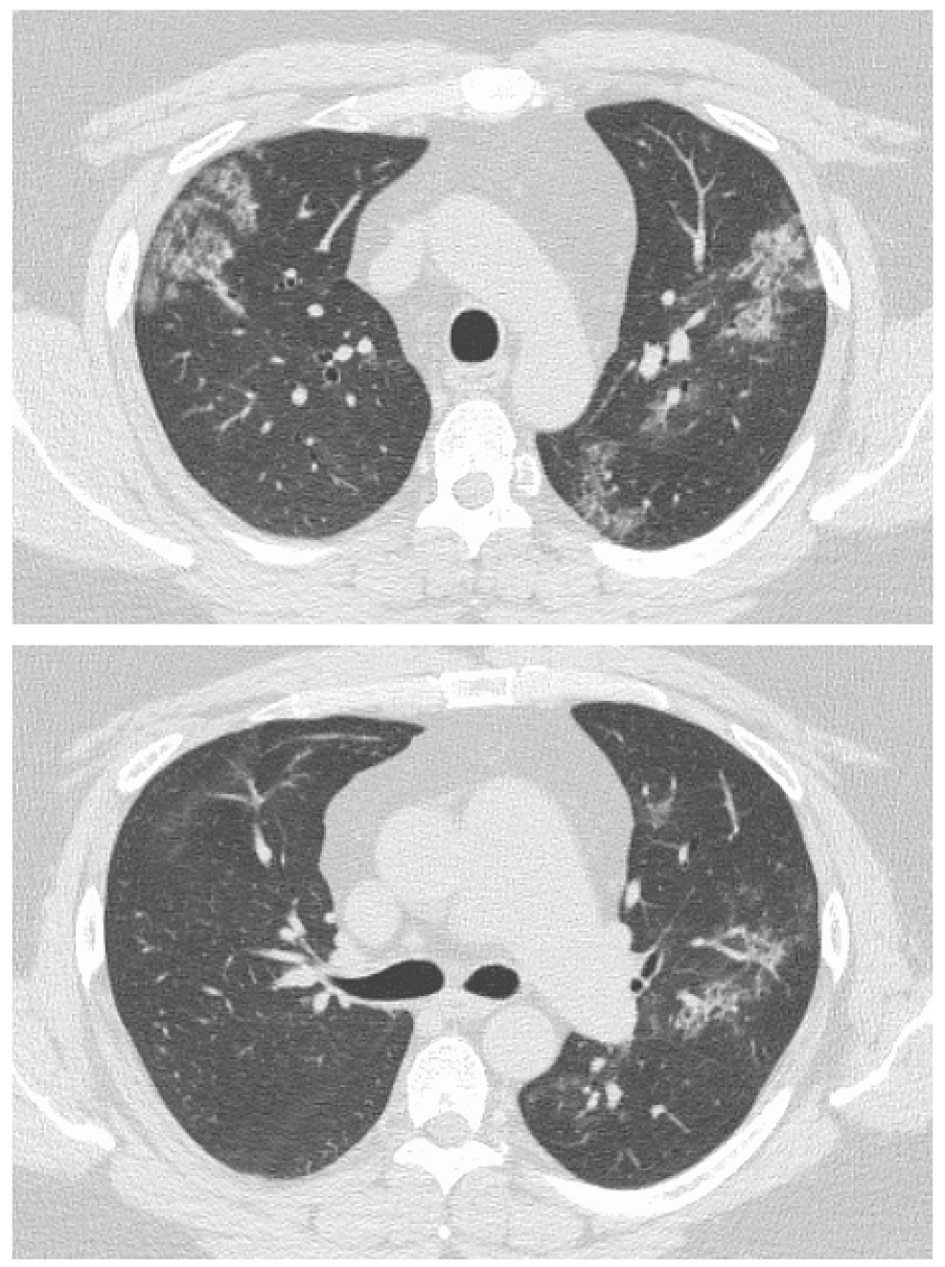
CT in July 2023 showed the appearance of central-side opacities. CT, computed tomography
